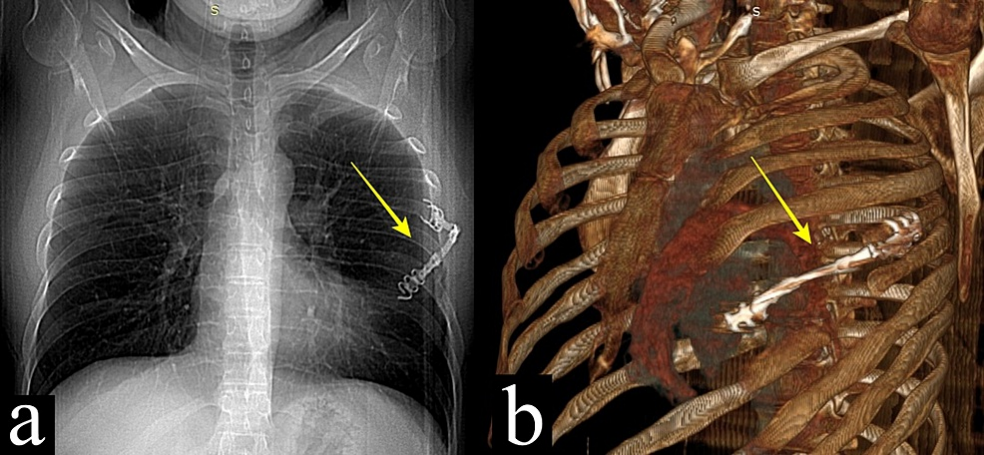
Postoperative chest x-ray (a) and 3D reconstruction of computed tomography (b) showing titanium bar reconstruction of the resected fifth rib (yellow arrow)
